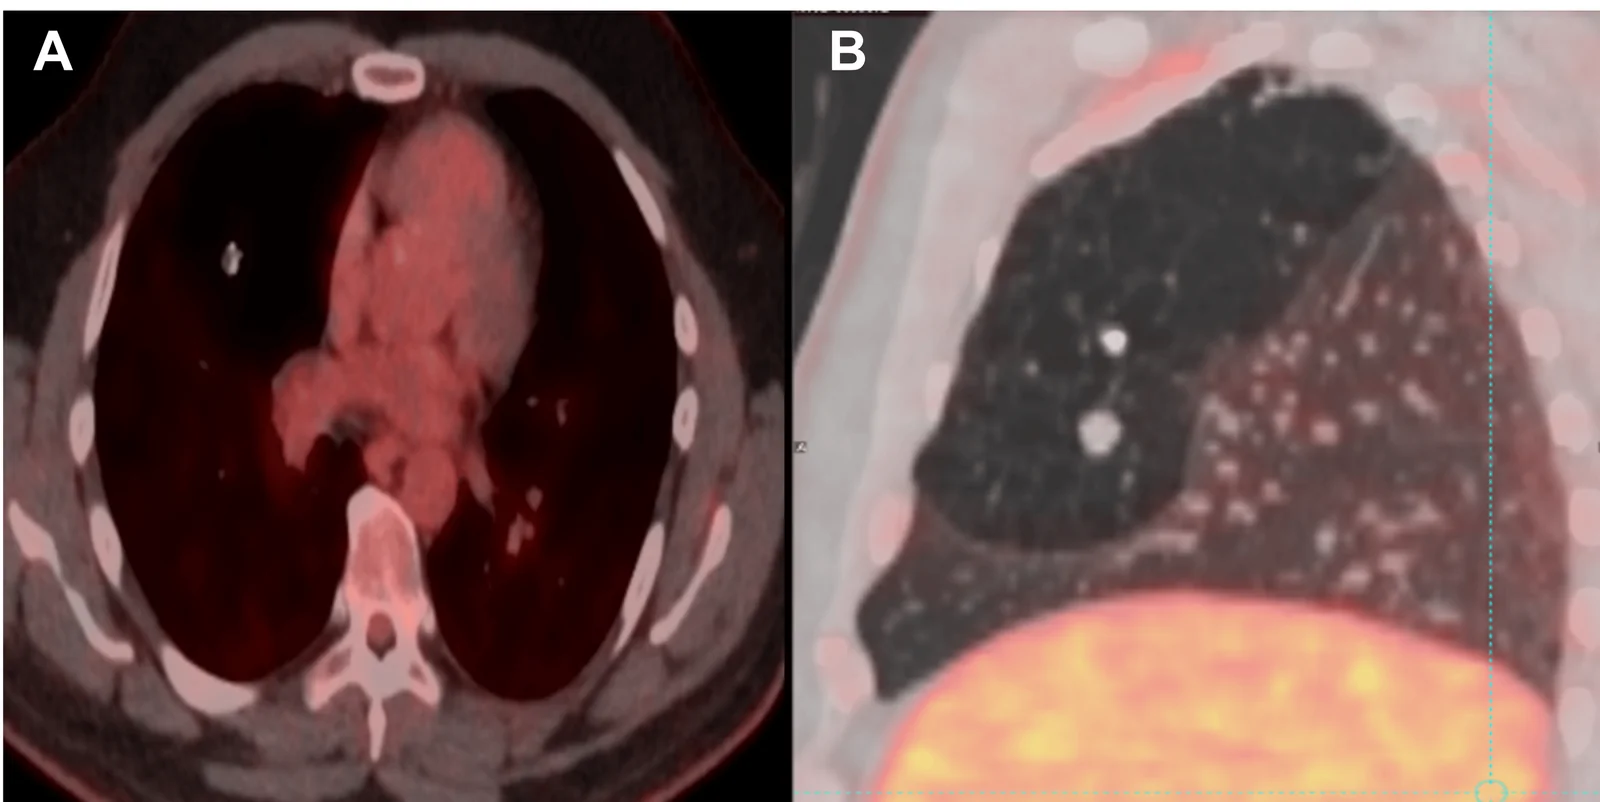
(A) Axial fused PET-CT demonstrating minimal FDG uptake within the right upper lobe cystic lesion. (B) PET-CT showing the cystic lesion with calcified nodules.
